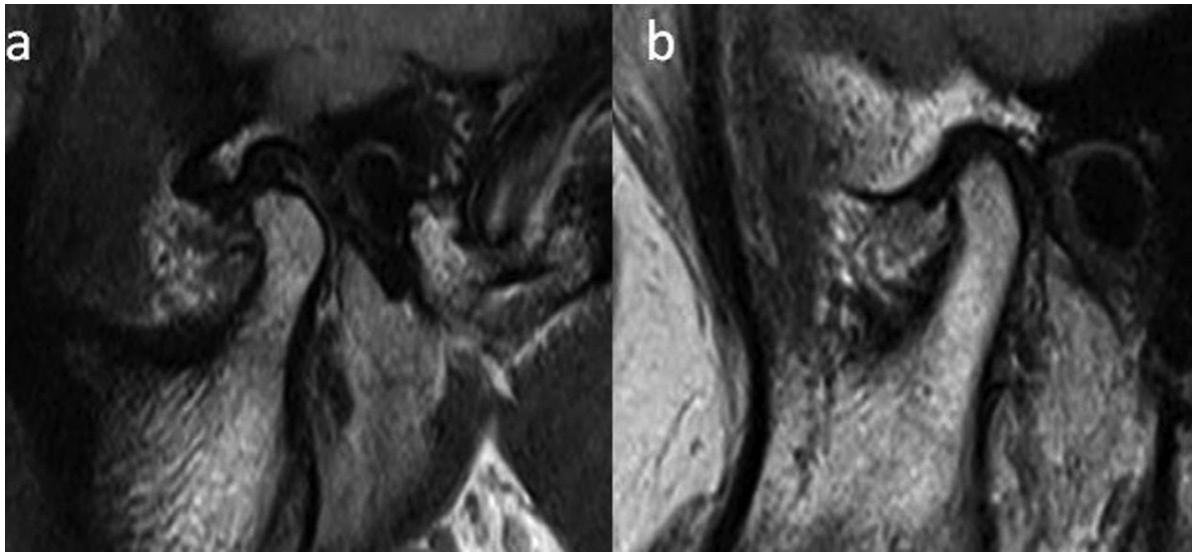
a): MRI of control showing ideal biconcave articular disc and normal joint space. b): MRI of OSMF patient showing thinning of disc and narrowing of joint space.
